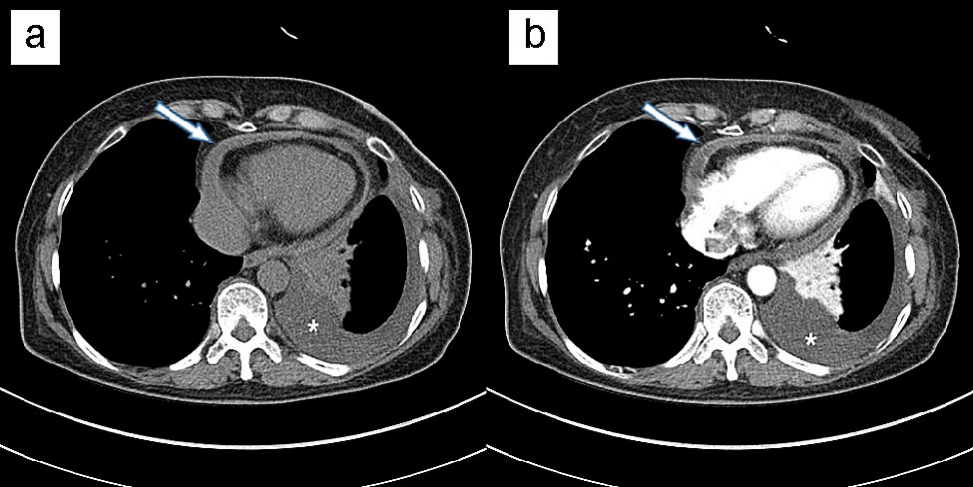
Chest computed tomography (CT) scan. (a) Non-enhanced image of the chest CT scan showing left-sided pleural effusion (asterisk) and pericardial thickening of up to 12 mm (white arrow). (b) Contrast-enhanced image showing pericardial effusions (white arrow) with pericardial rim enhancement suggesting effusive pericarditis. CT: computed tomography
